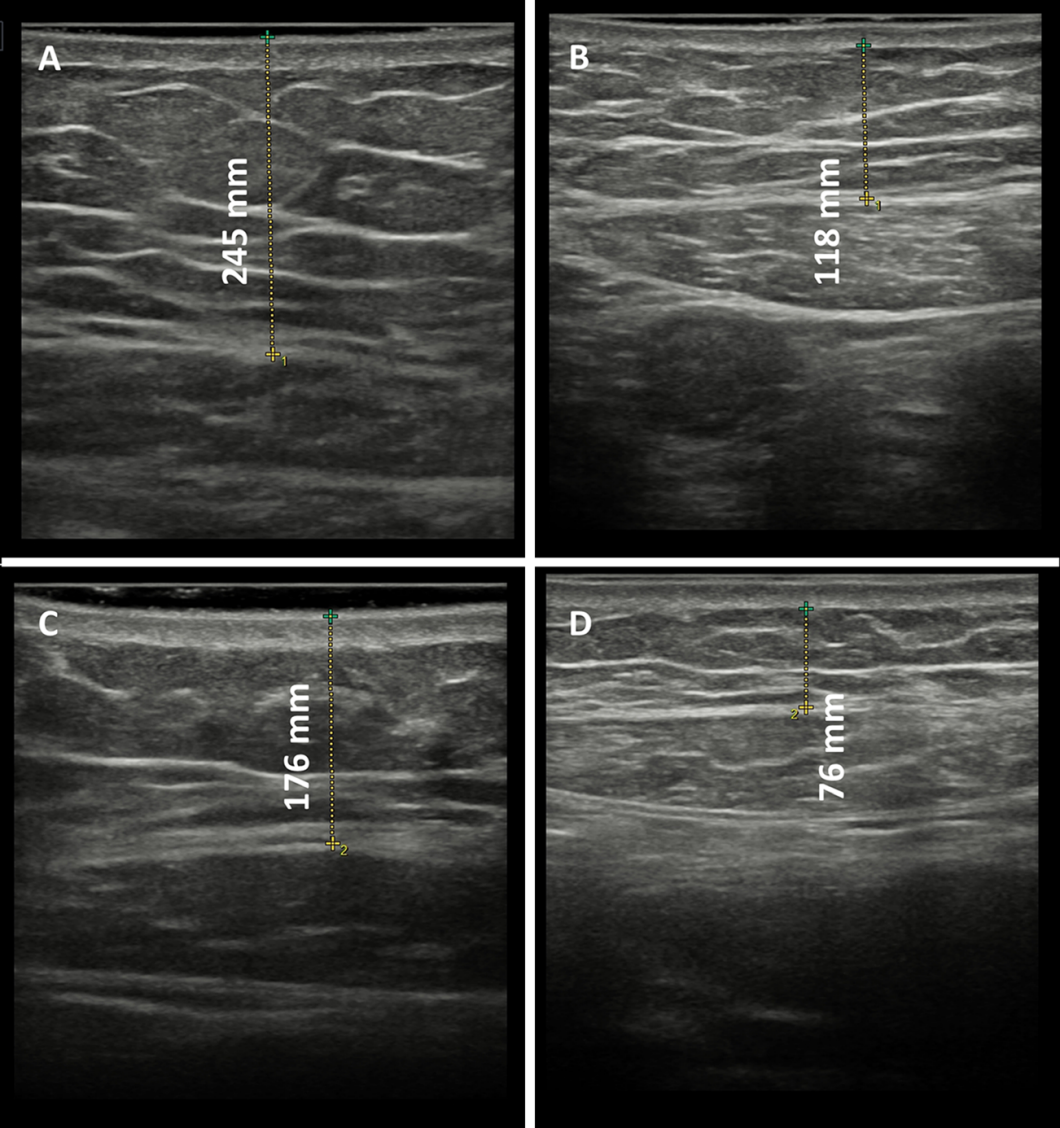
Ultrasound imaging of a 47-year-old woman who underwent four sessions in abdomen with the new non-invasive body contouring device Accent Prime X. A Infraumbilical thickness before treatment. B Infraumbilical thickness after treatment. C Supraumbilical thickness before treatment. D Supraumbilical thickness after treatment
